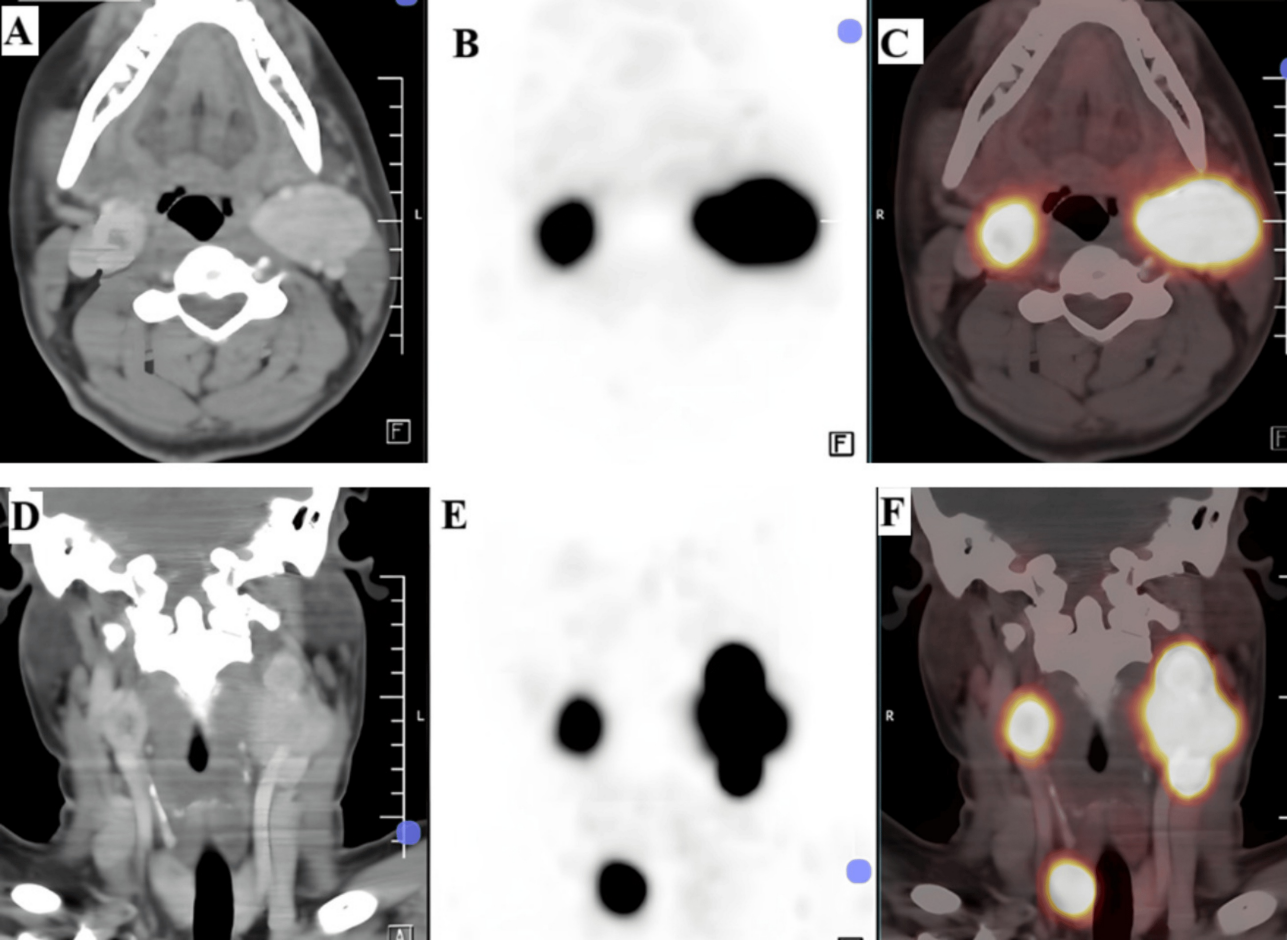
Ga-68-DOTANOC PET/CT demonstrating intense tracer avidity in bilateral carotid space lesions and additional foci (A) Axial contrast-enhanced CT image at the level of the carotid bifurcation demonstrating well-defined enhancing soft tissue lesions in the bilateral carotid spaces, splaying the internal and external carotid arteries. (B) Axial PET image showing increased radiotracer uptake corresponding to the carotid space lesions. (C) Axial fused PET/CT image demonstrating intense DOTANOC avidity in the bilateral carotid space lesions, with higher uptake in the left-sided lesion (SUVmax 54.3) compared to the right-sided lesion (SUVmax 28.36). (D) Coronal contrast-enhanced CT image demonstrating bilateral carotid space masses and an additional lesion lateral to the left external carotid artery. (E) Coronal PET image showing tracer-avid lesions corresponding to the carotid space masses and an additional inferior focus. (F) Coronal fused PET/CT image demonstrating intense DOTANOC uptake in the bilateral carotid space lesions, markedly increased avidity in the lesion lateral to the left external carotid artery (SUVmax 149.2), suggestive of high somatostatin receptor density, and additional DOTANOC avid focus in the right jugular bulb region (SUVmax 4.0) SUV: standardized uptake value
